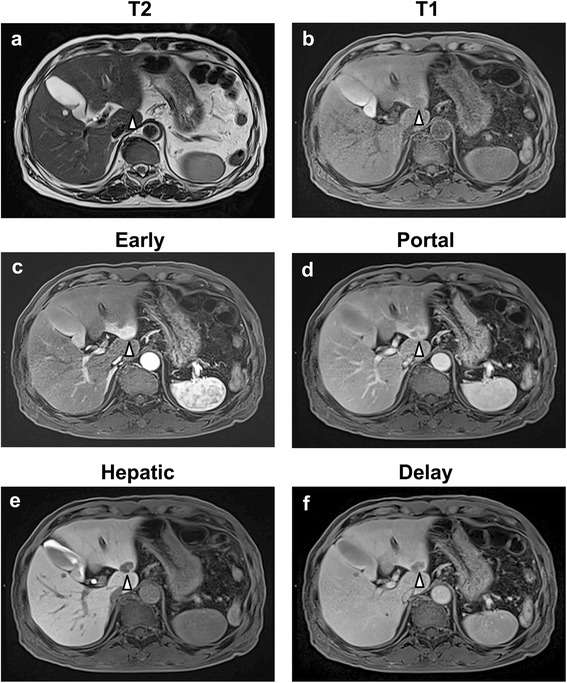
Magnetic resonance imaging (MRI) findings. a T2-weighted image with fat saturation showing a heterogeneous hyperintense mass in segment 2 of the right hepatic lobe. b T1-weighted image showing the lesion with a homogeneous low signal intensity. c–f Gadolinium ethoxybenzyl diethylenetriamine pentaacetic acid-enhanced magnetic resonance imaging findings. c Marked heterogeneous high signal intensity in the arterial phase of the dynamic gadolinium ethoxybenzyl diethylenetriamine pentaacetic acid-enhanced magnetic resonance imaging scan. d The signal intensity is relatively reduced in the portal venous phase, but slightly higher than in the surrounding liver parenchyma, with an enhanced vascular signal visible in the lesion. e The signal intensity of the tumor is lower at the parenchymal phase than in the surrounding liver parenchyma. f A lack of gadolinium ethoxybenzyl diethylenetriamine pentaacetic acid-enhanced magnetic resonance imaging uptake is noted in the hepatobiliary phase at 40 minutes postinjection. *Arrowheads* denote tumor lesion in segment 2 of liver
